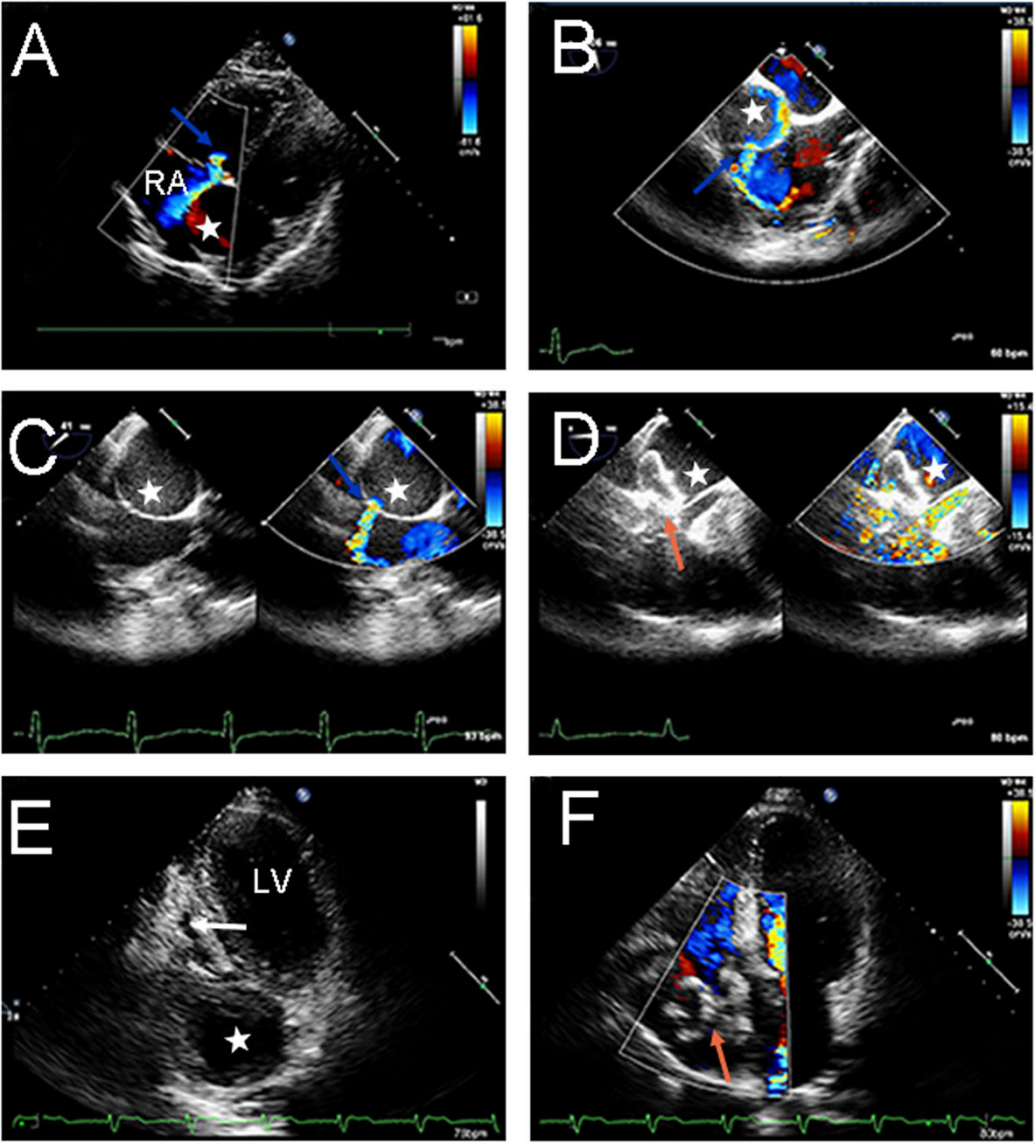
Echocardiogram before, during and after 3 months of operation findings: a, b Pre-operation echocardiography demonstrated a giant right coronary artery aneurysm (65*48 mm) (white star) with a fistula to the RA (blue arrow); c, d Intraoperative TEE showed AAU and fistula. A 30 mm ASD occluder (red star) was placed; e, f 3 months post-operation, TTE revealed a residual aneurysm of about 42*42 mm, mural thrombosis in the aneurysm, and no residual shunt between RCA and RA was found. The visual segment of the right coronary artery was still dilated (white star). (RA right atrium; TTE transthoracic echocardiography; ASD atrial septal defect; RCA right coronary artery; RA right atrium; LV Left ventricle)
